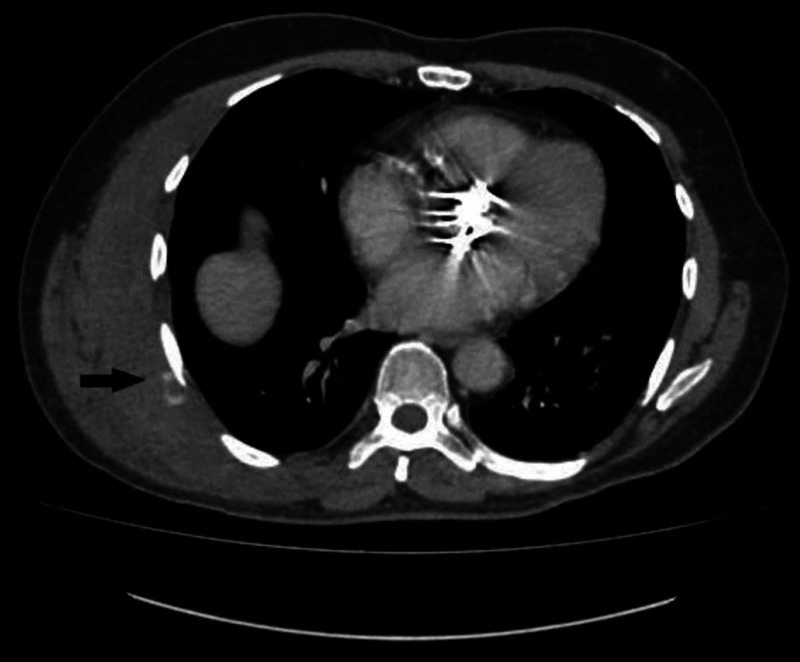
Contrast-enhanced CT chest demonstrates a large chest wall hematoma on the right lateral chest wall. The arrow in black highlights the active bleeder.
